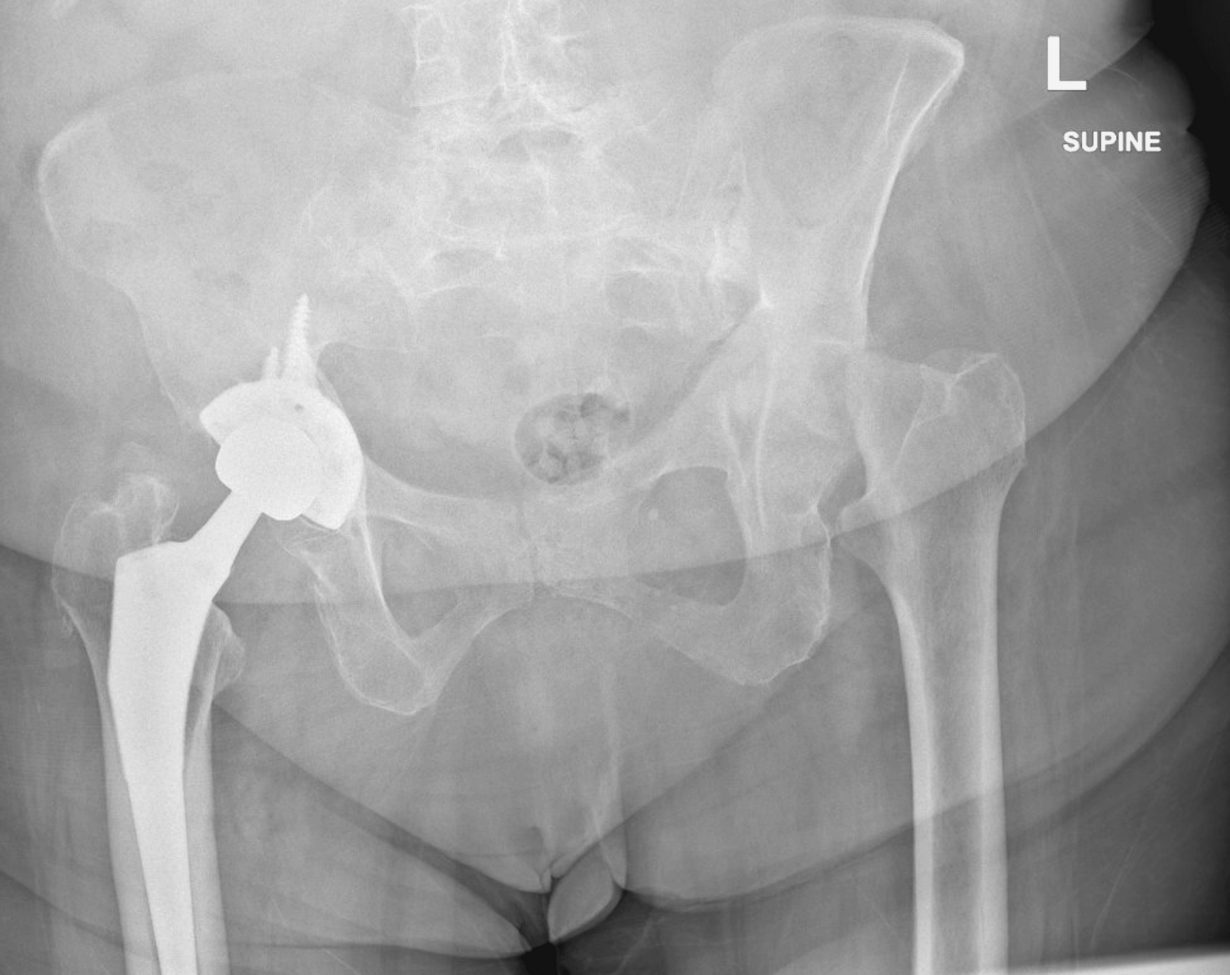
Preoperative AP X-ray of the pelvis AP: anteroposterior
